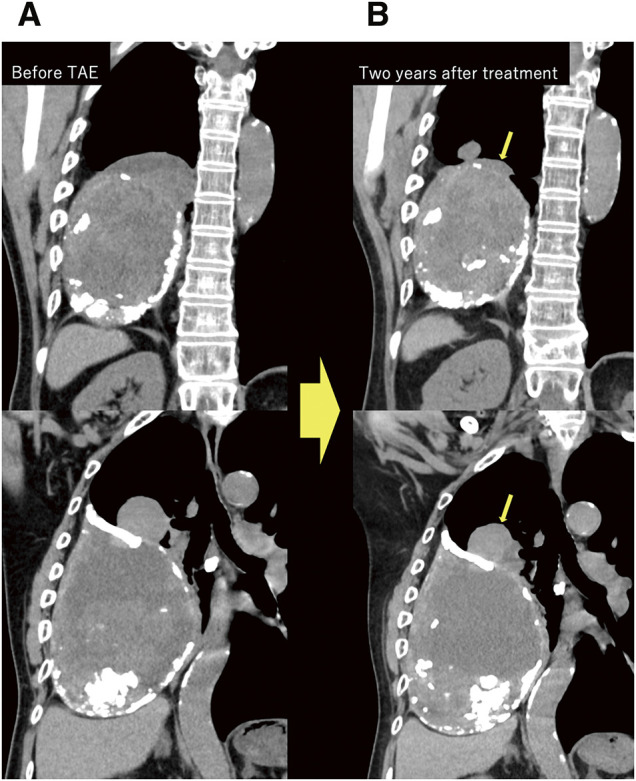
Coronal chest CT images before TAE (A) and 2 years after treatment (B). The cranial protrusions demonstrate significant size reduction at follow-up (arrows).
